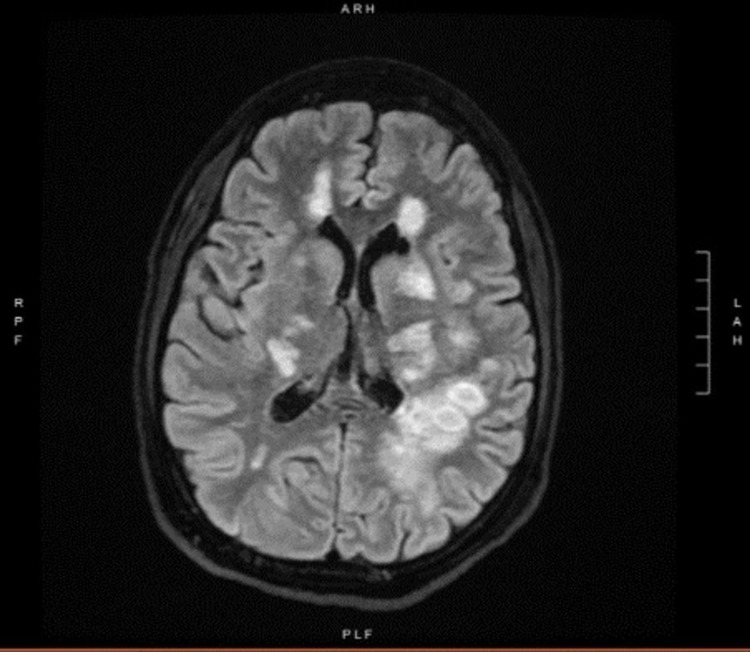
MRI T2-weighted image showing multiple ring-enhancing lesions. MRI, magnetic resonance imaging
